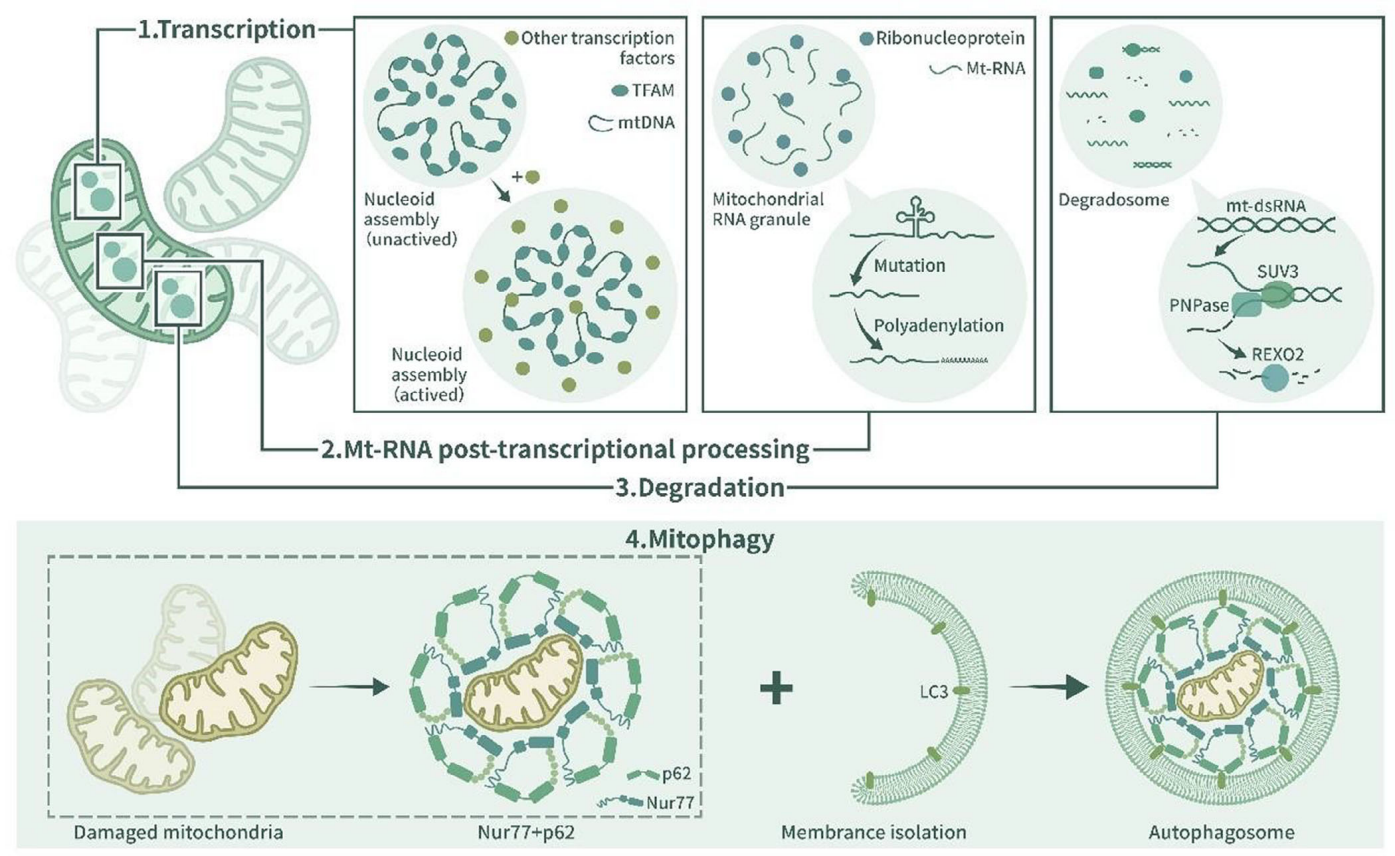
Mitochondrion‐related liquid–liquid phase separation. (1) Phase separation mediates the self‐assembly of mitochondrial nucleoids (mt‐nucleoids): mt‐nucleoids are formed by the phase separation of mitochondrial DNA (mt‐DNA) with TFAM. The components that make up the mt‐nucleoids change when nucleoid formation is in different stages. Mt‐nucleoids recruit factors including transcription initiation complexes, elongation complexes and termination factors through phase separation. (2) Phase segregation mediates the formation of mitochondrial RNA granules (MRGs): immature single‐stranded mRNA from mt‐nucleoids undergoes processing, maturation, polyadenylation and stabilization in MRGs. Mature mRNA transcripts are transferred from MRGs. (3) Phase separation mediates the formation of mitochondrial degradosomes: The degradosome is a site where mt‐dsRNA is degraded by SUV3, PNPase, and REXO2. SUV3 mediates double‐stranded RNA segregation, PNPase hydrolyses single‐stranded RNA into fragments, and REXO2 degrades RNA sequences comprising fewer than four nucleotides. (4) Phase segregation is involved in mitophagy: Nur77 undergoes phase separation with p62∖SQSTM1, thereby isolating damaged mitochondria and initiating mitophagy by binding LC3 on the autophagosome membrane.
